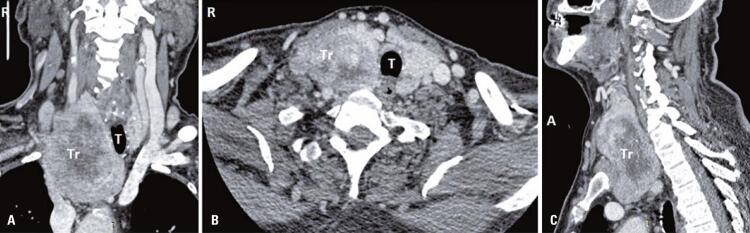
Patient’s coronal (A), axial (B), and sagittal (C) neck computed tomography images with contrast. Deviated trachea, obliterated internal jugulary vein and substernal extention of the thyroid can be observed
